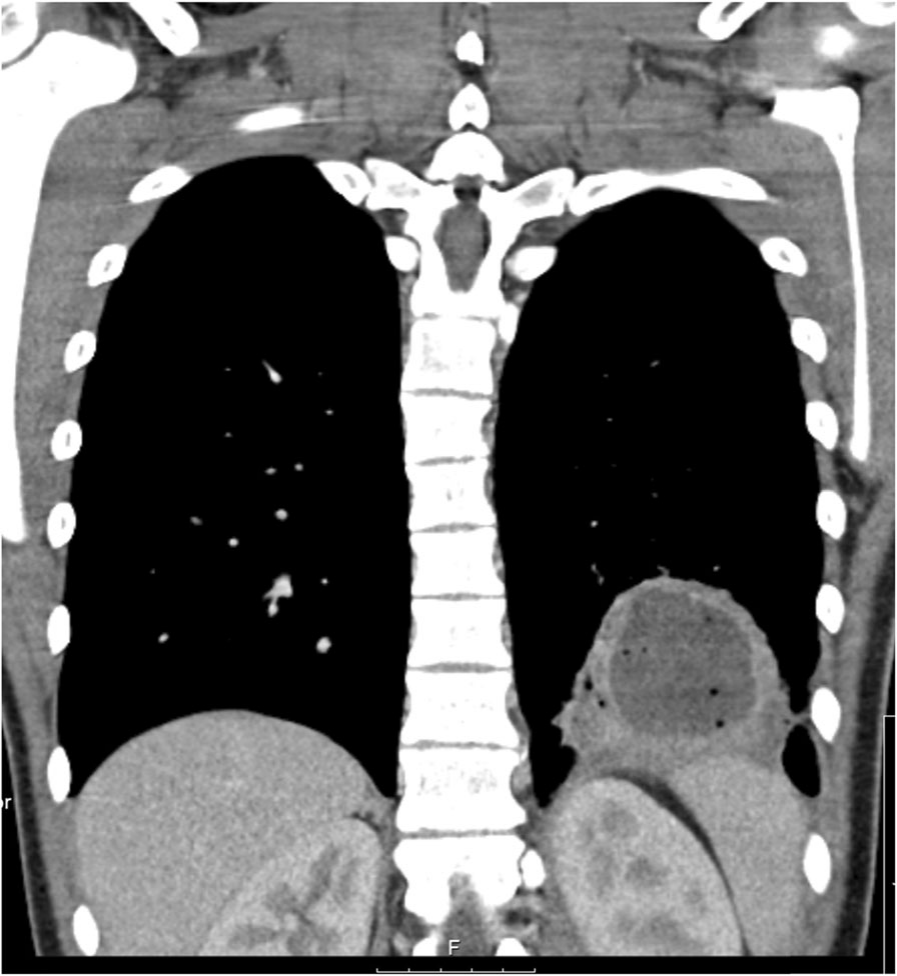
Frontal plane of the chest CT scan
